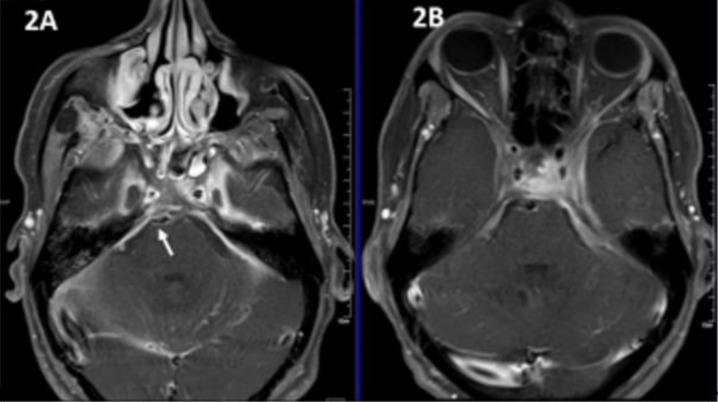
Post-contrast T1 weighted fat-saturated magnetic resonance imaging (MRI) images showing florid sinusitis with contiguous abnormal enhancement noted in bilateral cavernous sinuses and dura (arrow) (2A). Post-treatment MRI showing resolution of sinus inflammation with residual soft tissue in the left cavernous sinus and associated dural enhancement (2B).
